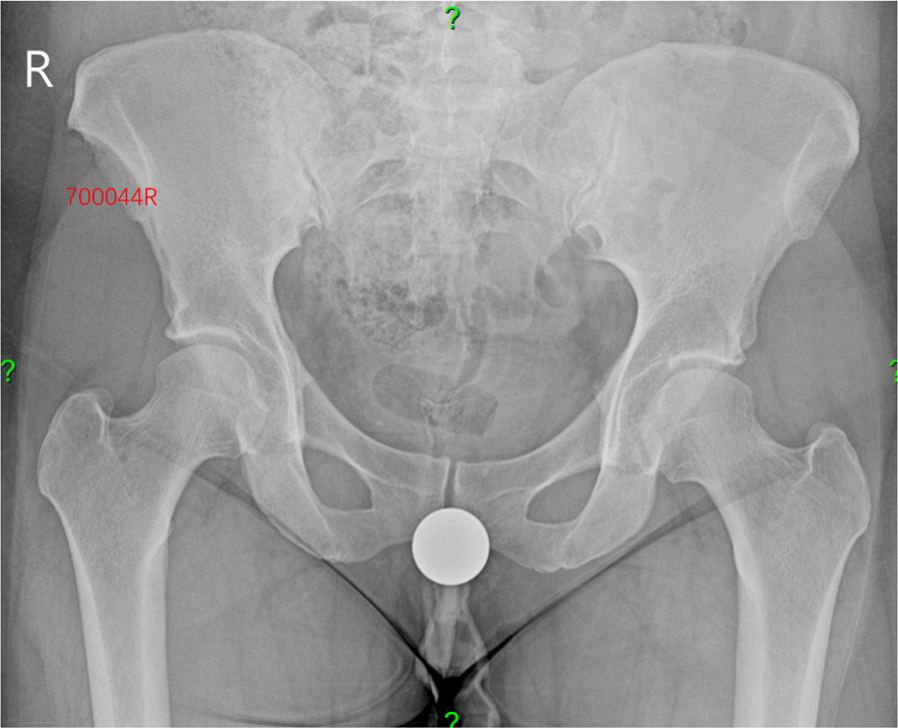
Image of blinded hip radiograph. The medical reference number and the side under consideration is in red
